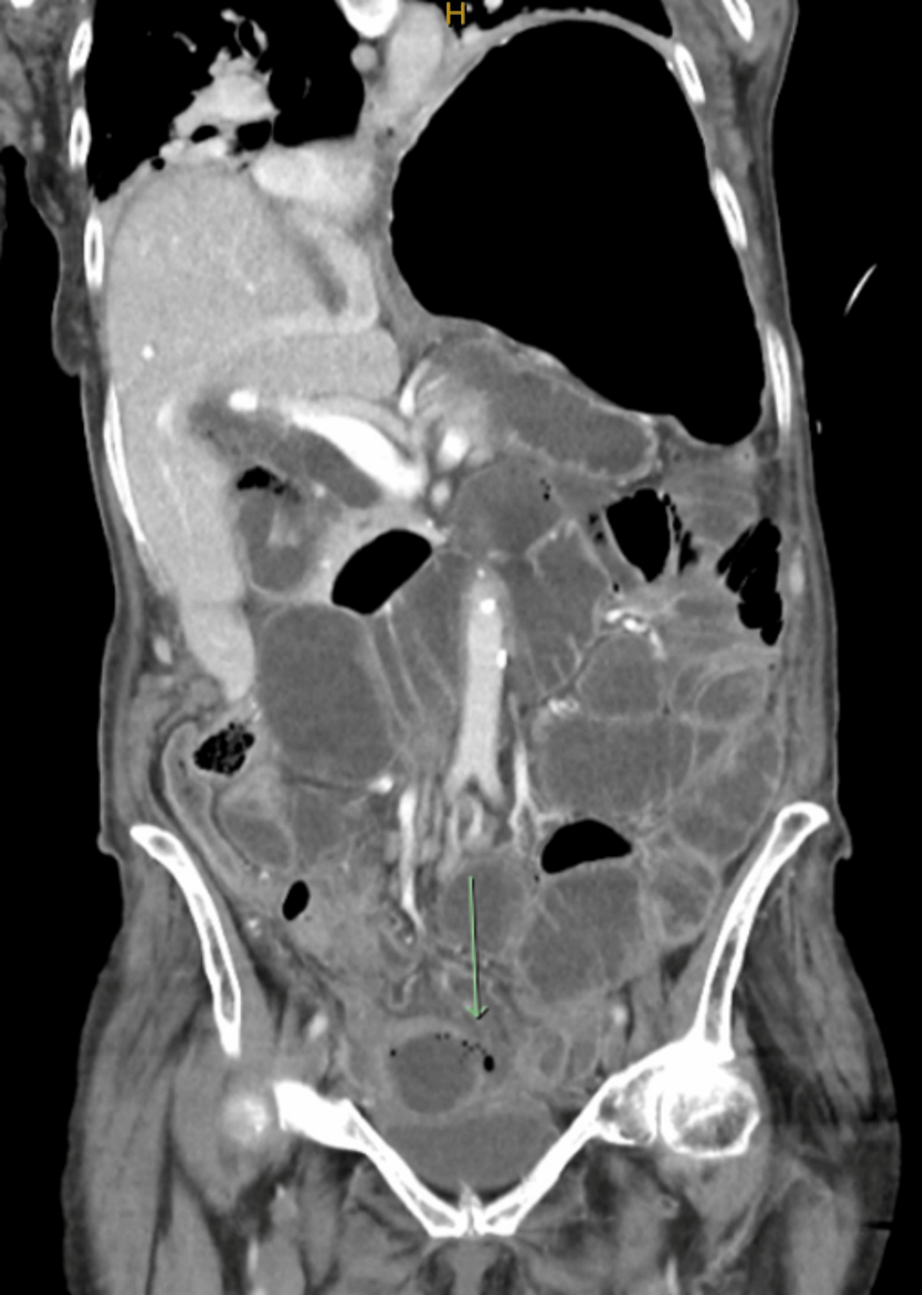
Coronal view of computed tomography (CT) abdomen The image shows dilated small bowel loops with evidence of small bowel perforation (green arrow) and resultant small-volume pneumoperitoneum.
